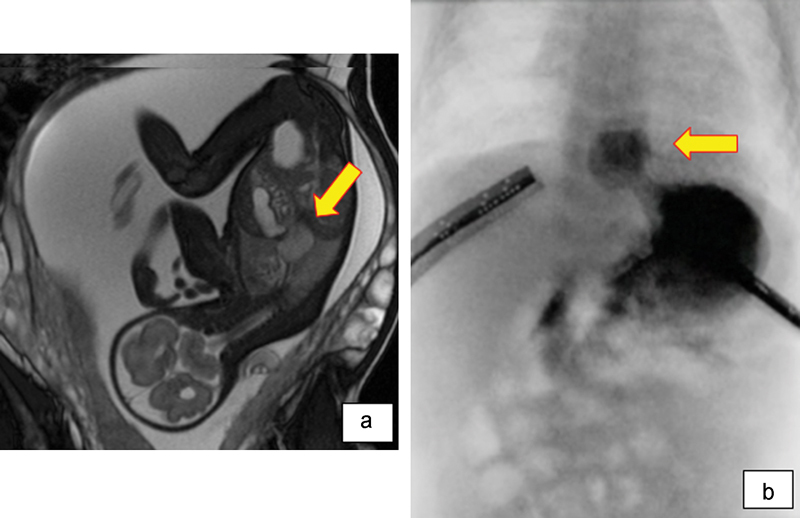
(a) Fetal magnetic resonance imaging shows a cystic lesion with slightly high intensity in true FISP and HASTE mode (arrow). (b) Esophagography shows a hiatal hernia and gastroesophageal reflux (arrow).
